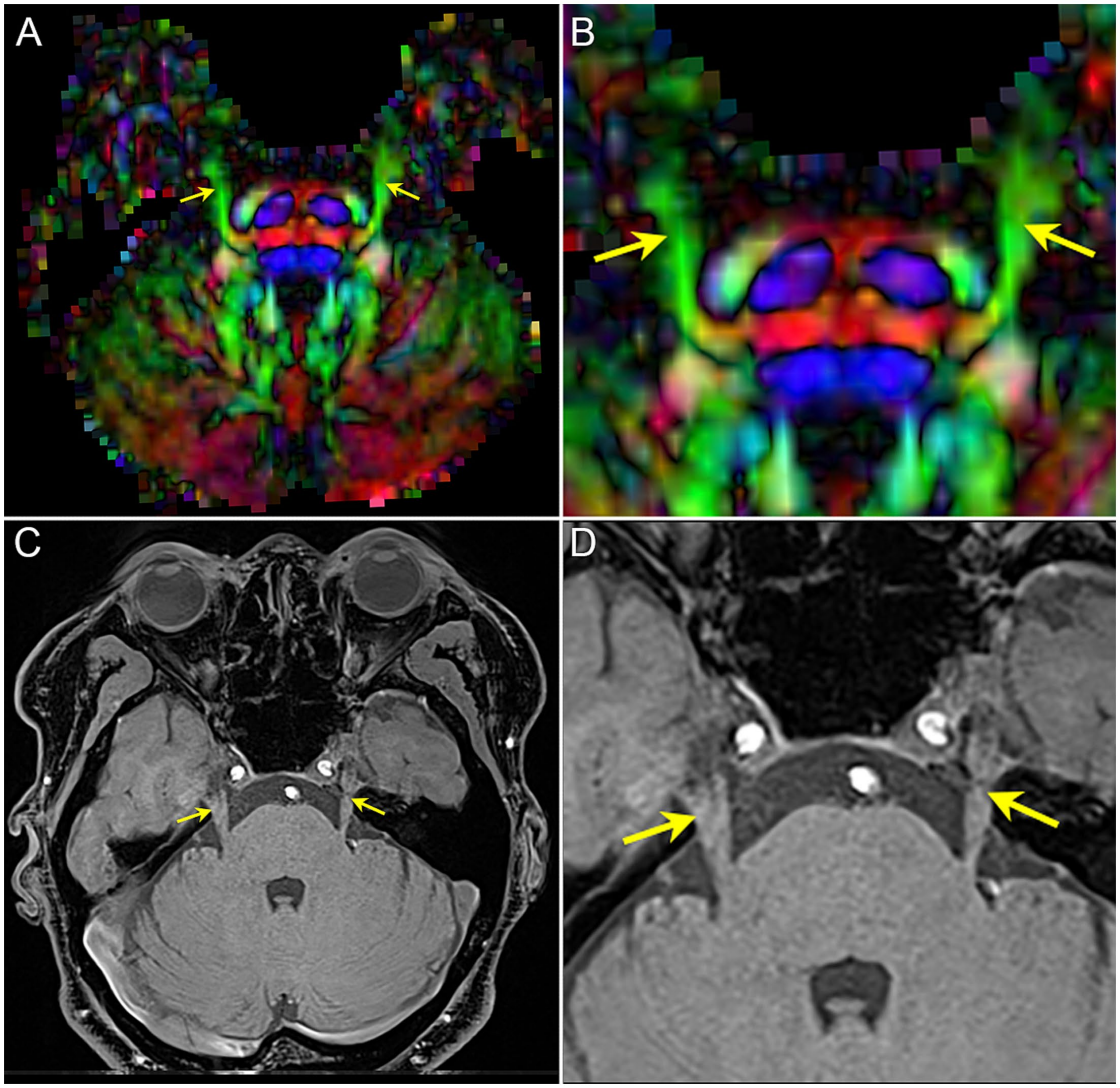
The image shows the bilateral CNV tracts in DTI and anatomical MRI. (A,B) the FA map of DTI image, image B is the focal magnification of (A); (C,D) T1-weighted image of MRI, image D is the focal magnification of (C). Yellow arrow: the bilateral CNV.
